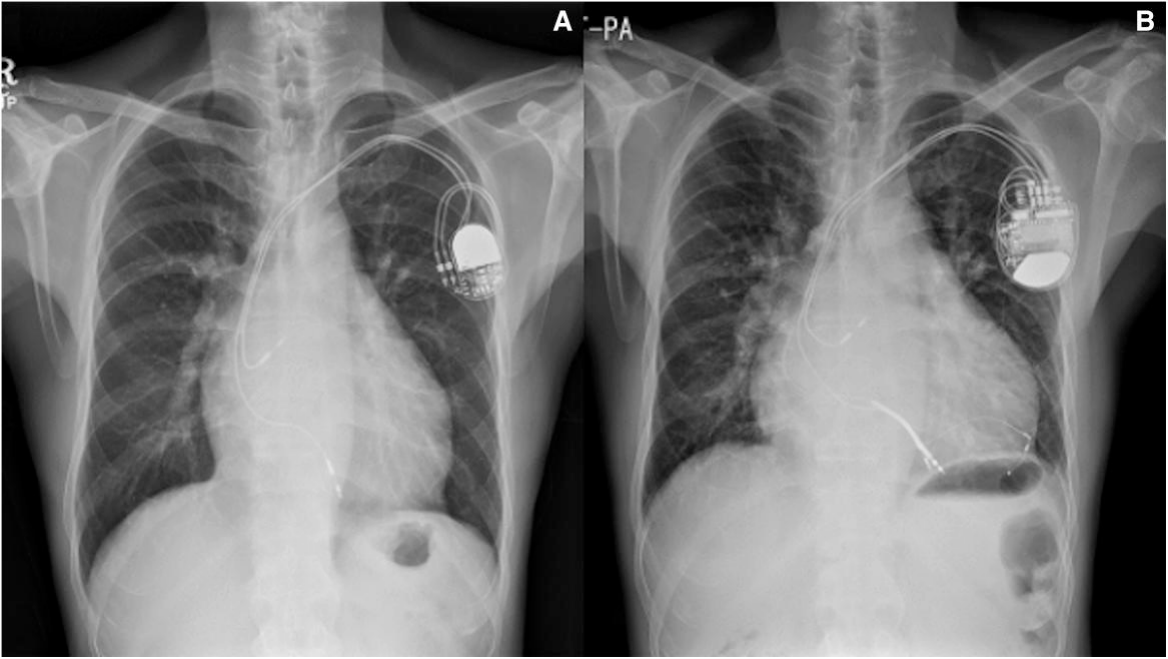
Chest radiography of a 57-year-old male patient with ccTGA, post-implantation of a dual-chamber PPM for complete heart block with mild cardiomegaly *(A)* and worsening sRV failure over time *(B)* despite upgrade to CRT.
